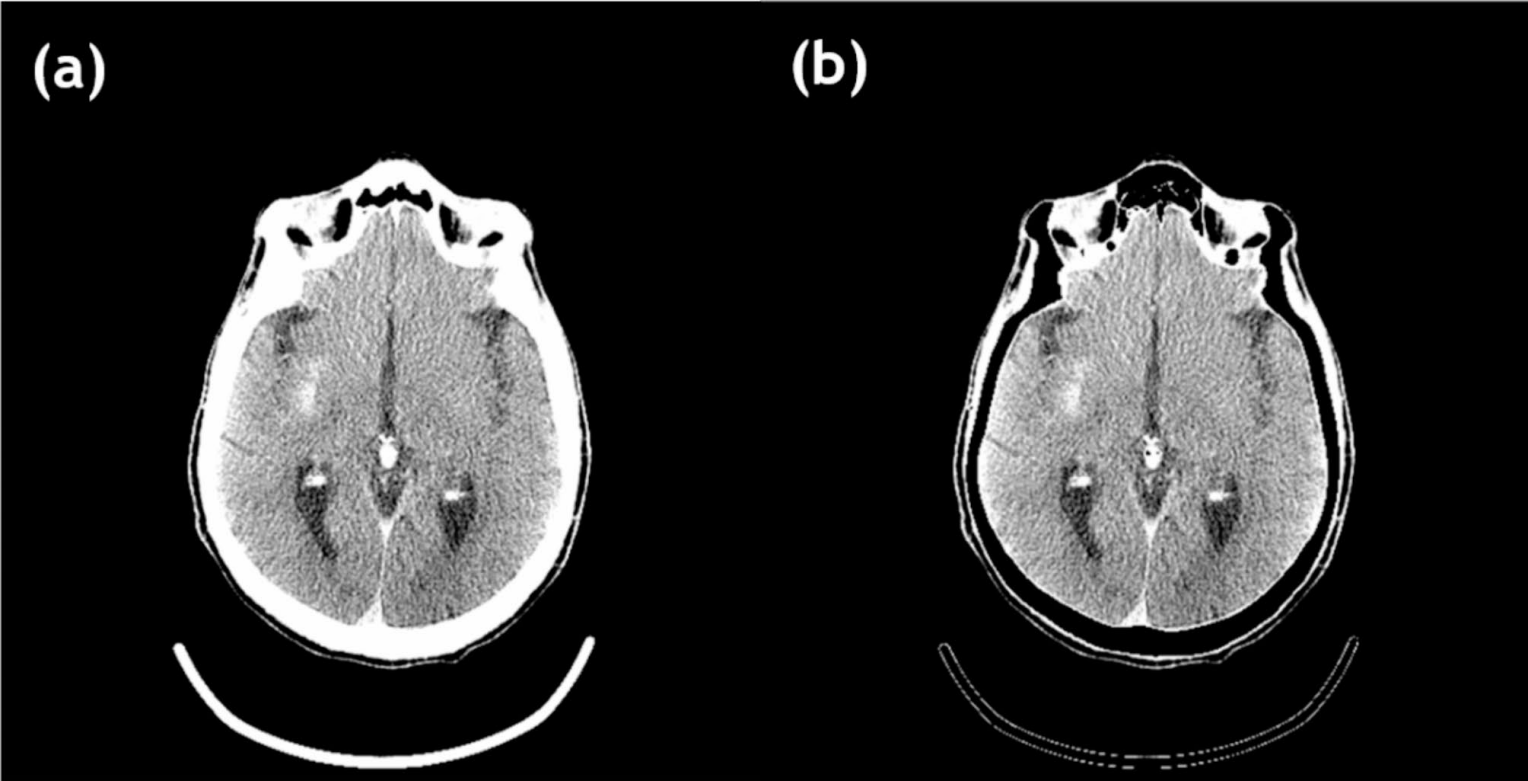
The human brain image from PCD-CT (a) and the images applied bone removal method (b). The threshold was set as 0.3. The region of bone in the human brain was removed.
